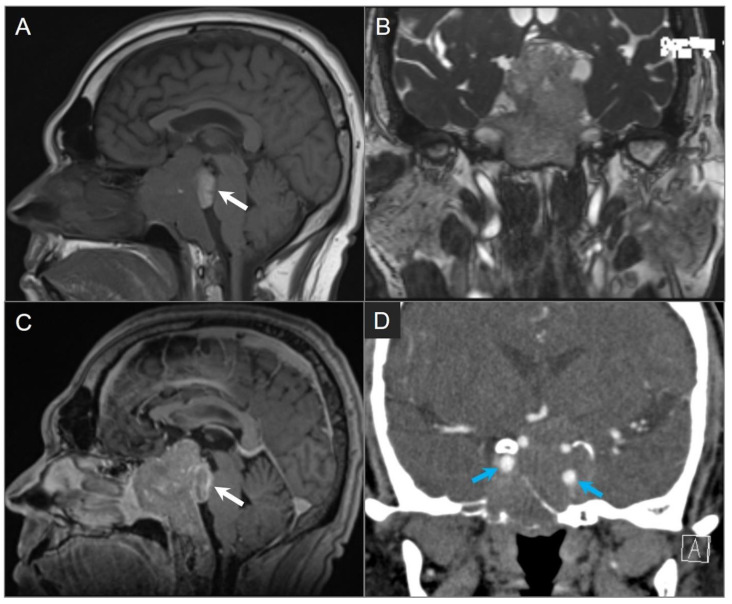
Giant invasive macroadenoma. (A) Sagittal noncontrast T1W, (B) coronal postcontrast CISS, and (C) sagittal postcontrast T1W MR images show tumor invading the clivus, cavernous sinuses, and posterior sinonasal cavity. Probably hemorrhagic T1 hyperintense component of the tumor extends into the prepontine cistern and compresses the pons (white arrow) with mass effect on the optic chiasm and third ventricle. (D) Coronal CTA shows encasement of the left greater-than-right cavernous internal carotid artery without significant vascular narrowing (blue arrows).
